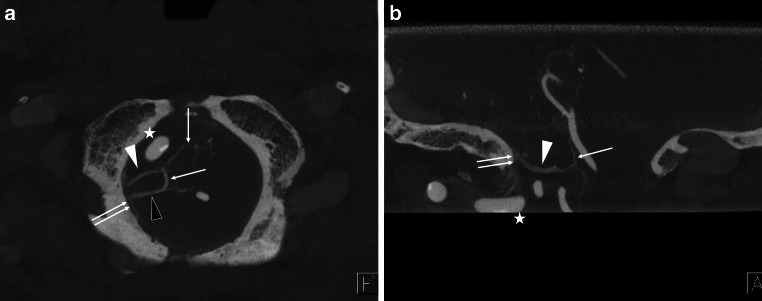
A representative case of the marginal sinus (MS) bridging vein (BV). Axial (a) and coronal (b) view of the slab MIP images from contrast-enhanced cone-beam CT show that the transverse medullary vein (*arrow* in (a)) and lateral medullary vein (*arrow* in (b)) drains into the MS (*double*
